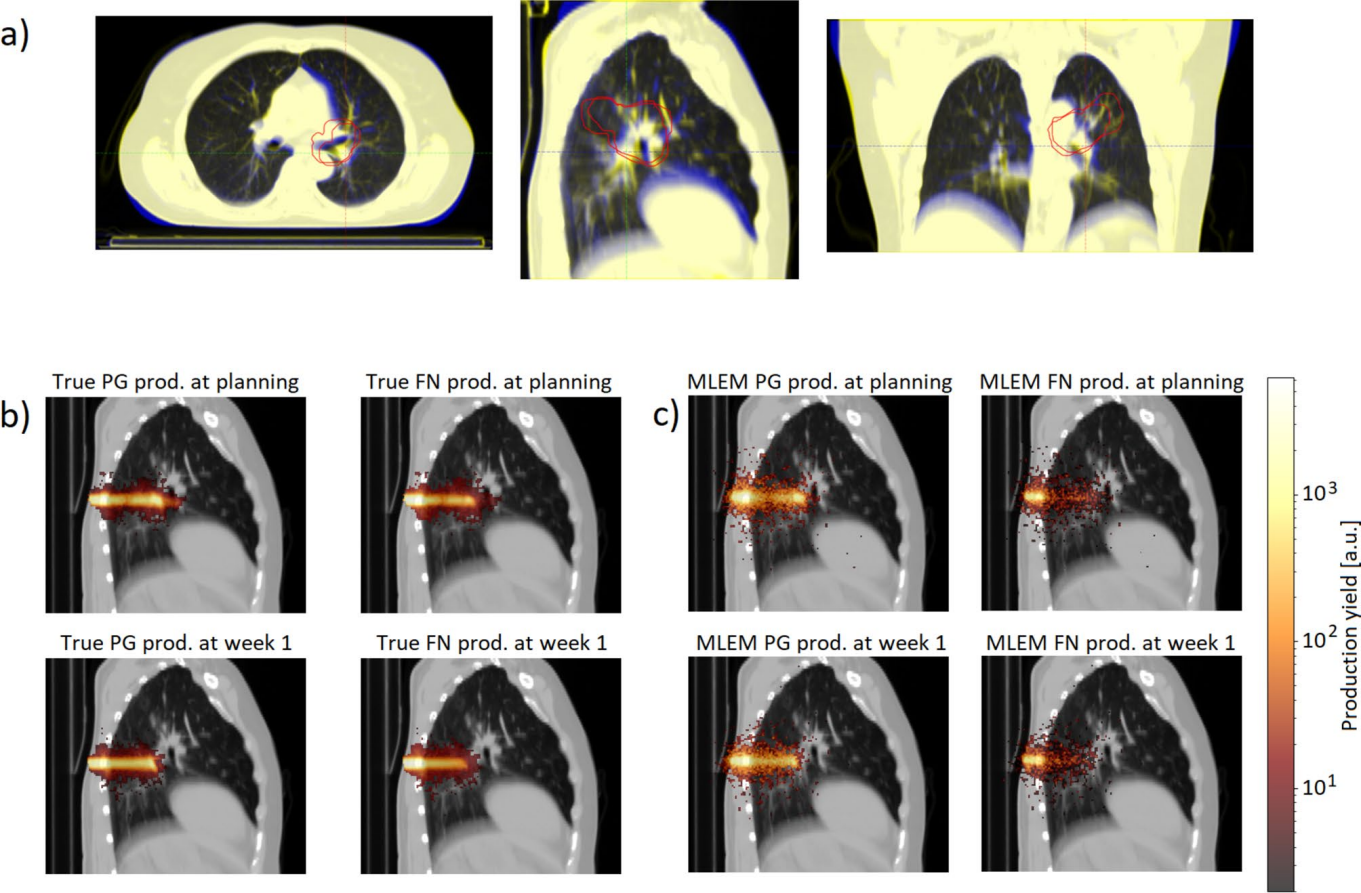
Example of treatment deviation caused by anatomical changes. (a) The axial, sagittal and coronal plane of the example patient with the average intensity projection of the 4D planning CT (yellow) overlaid on the repeat CT (blue). The target volume contoured by the treating oncologist on both CTs are visible as red contours. Both a shift and deformation of the target volume with corresponding change in tissue densities can be observed. The lungs are larger on the planning compared to the repeat CT indicating a shallower breathing pattern during acquisition of the latter. (b) PG and FN production, both from MC truth and reconstructed with MLEM, from the planning CT (top) and when produced in the week 1 CT, overlaid on the planning CT (bottom).
